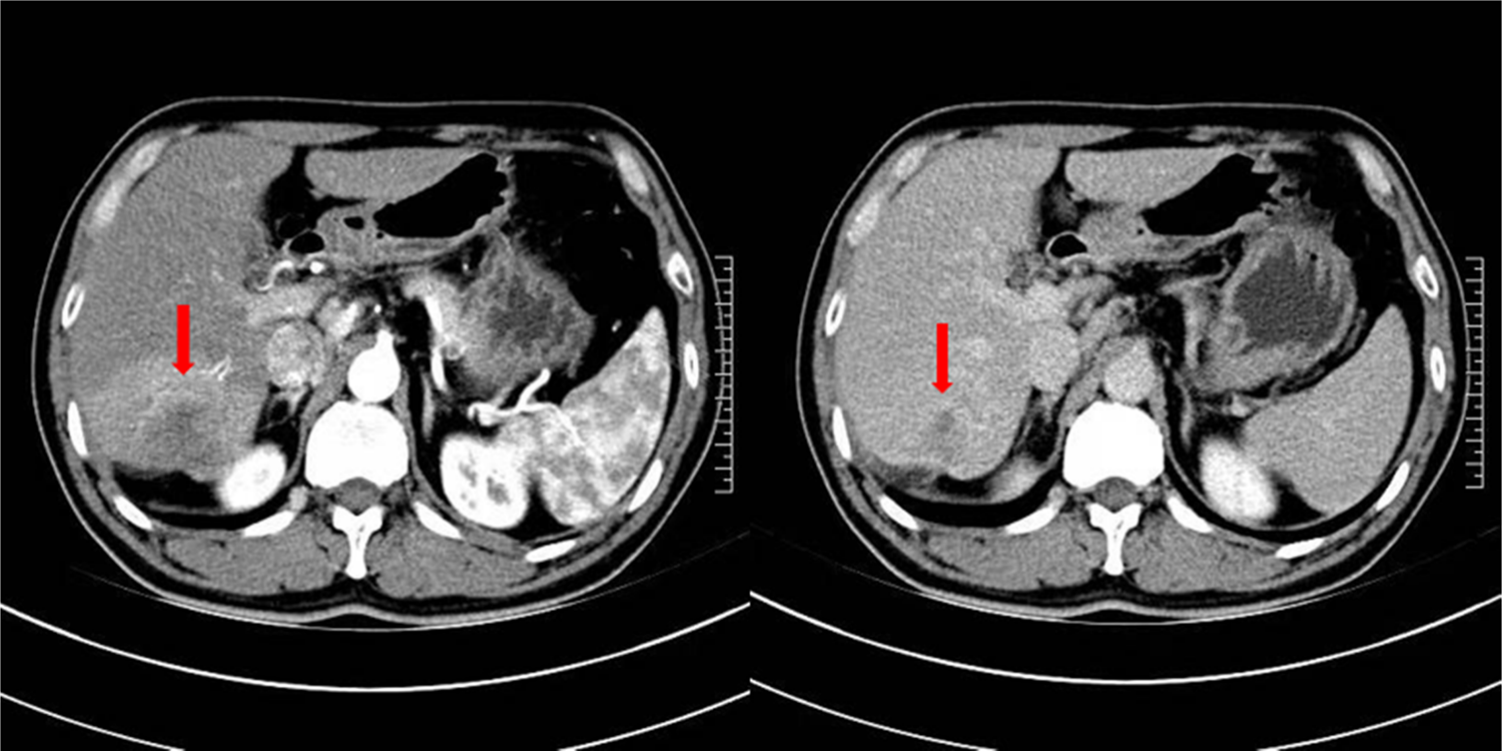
CT-scan showing the size of lesion. The arrow points to a lesion of 5.5 cm × 5.1 cm.
